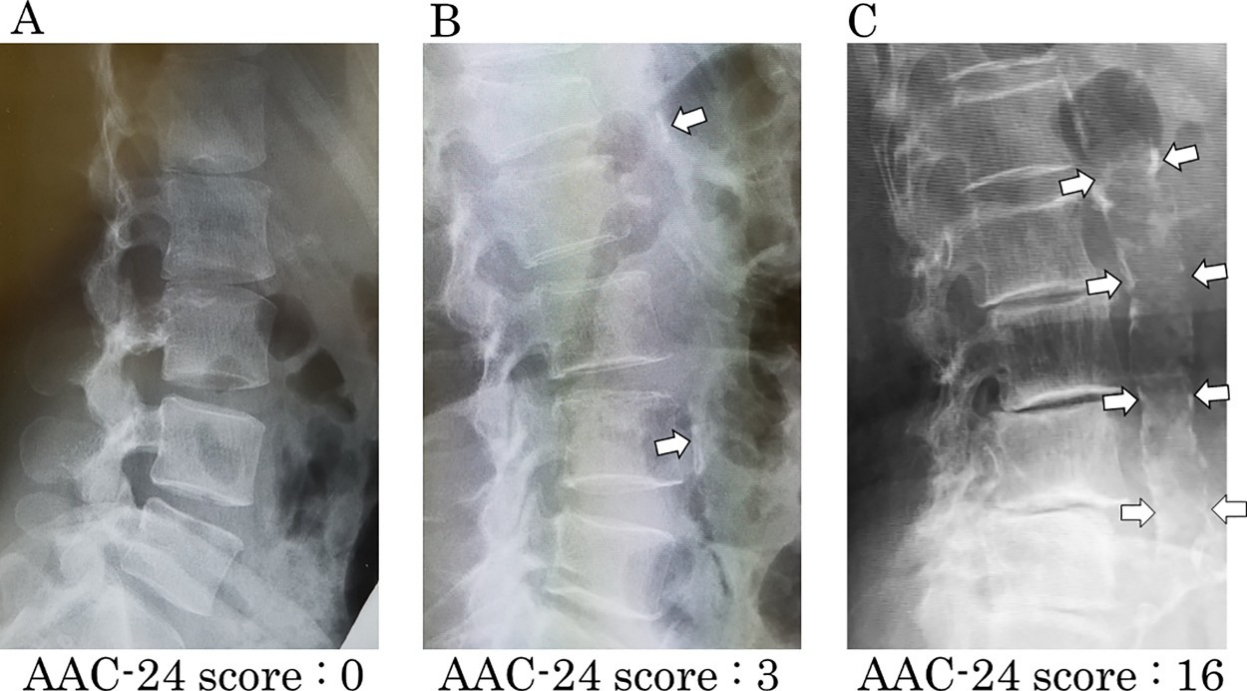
Radiographic images with (A) no abdominal aortic calcification (AAC), (B) moderate AAC (AAC-24 score = 4), and (C) moderate AAC (AAC-24 score = 16). AAC is indicated by white arrows.
